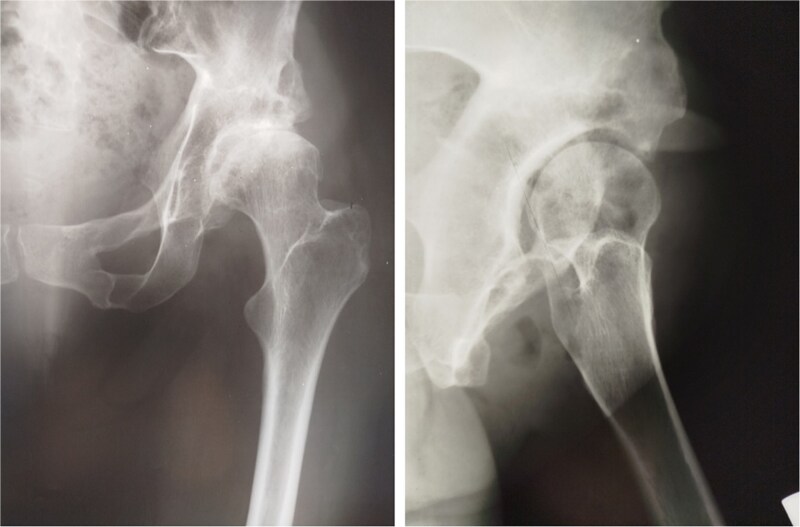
Postoperative radiographs of the pelvis 20 years after pelvic osteotomy, showing signs of moderate osteoarthritis in the left hip (Tönnis Grade III), and radiographic evidence of acetabular retroversion (ischial spine sign and posterior wall sign).
